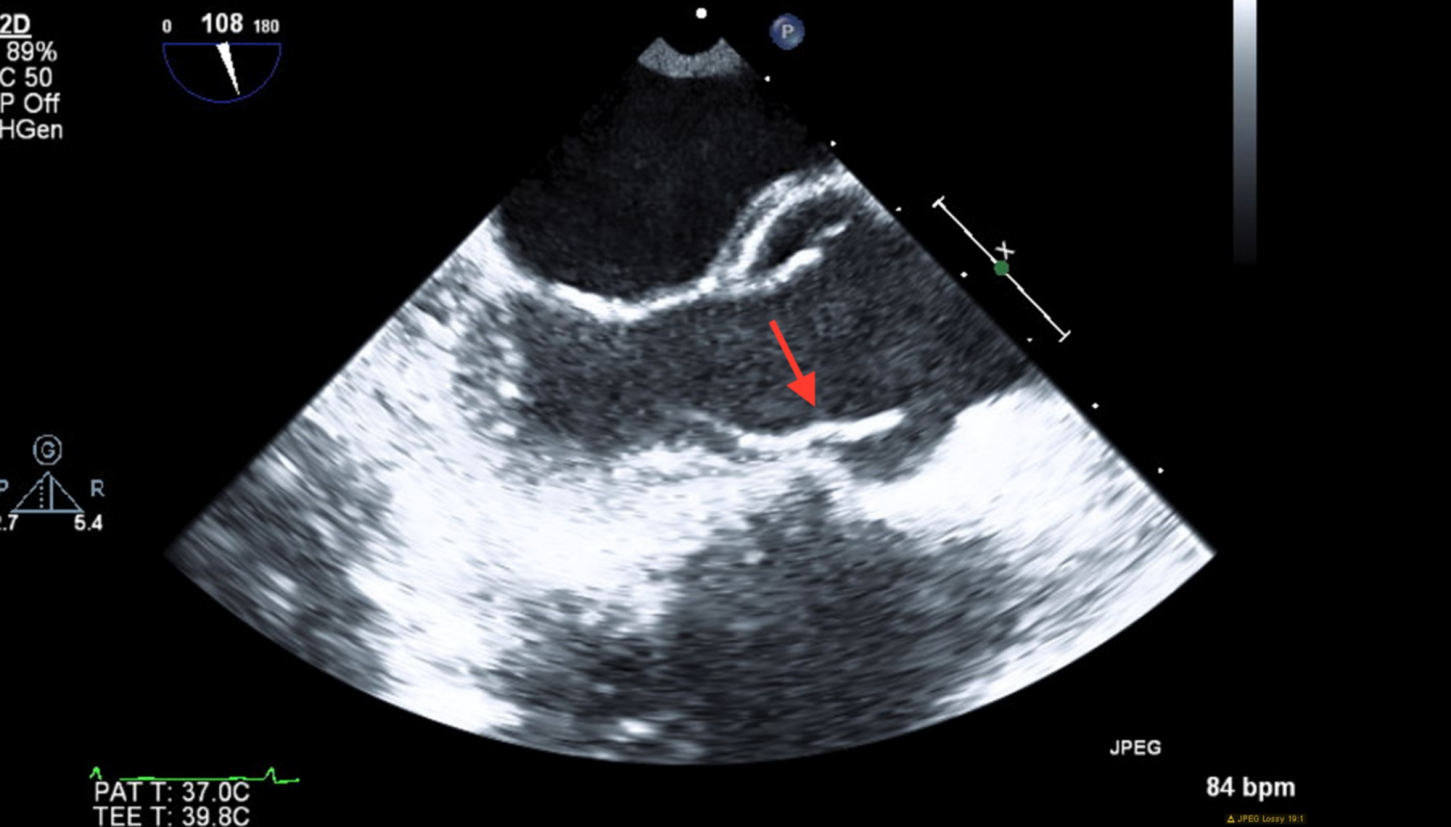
TEE depicting filiform lesion noted on aortic valve leaflet tips most likely LE. TEE, transesophageal echocardiogram; LE, Lambl's excrescence
